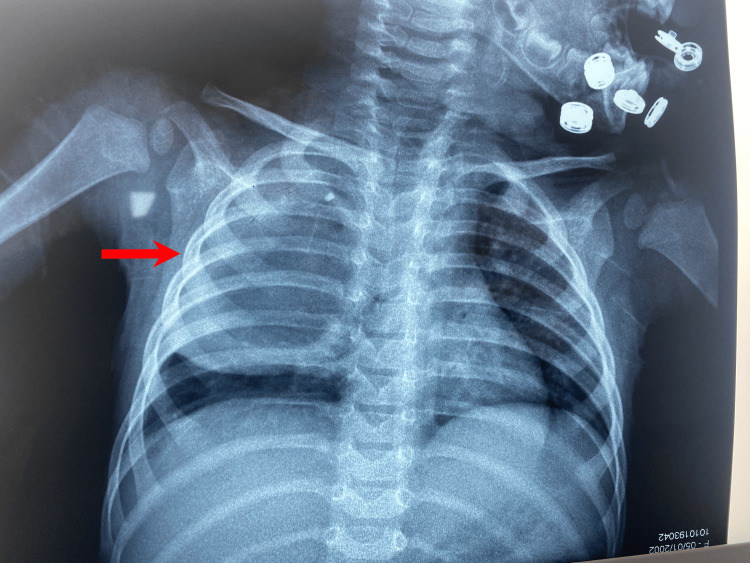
Frontal chest X-ray. The frontal chest X-ray reveals a well-defined opacity of water density, extending to the upper two-thirds of the right lung, without evidence of mediastinal displacement.
